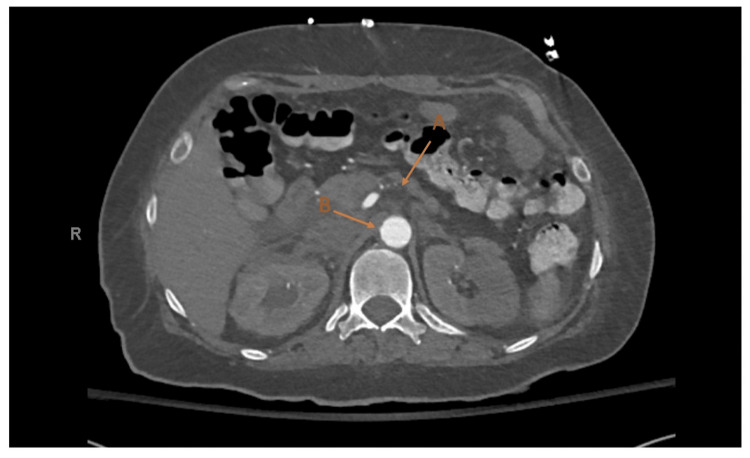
SMA occlusion with extension into the abdominal aorta on CT abdomen and pelvis with contrast A: SMA occlusion with lack of contrast. B: Aorta with contrast as comparison. SMA: Superior Mesenteric Artery; CT: Computerized Tomography.
